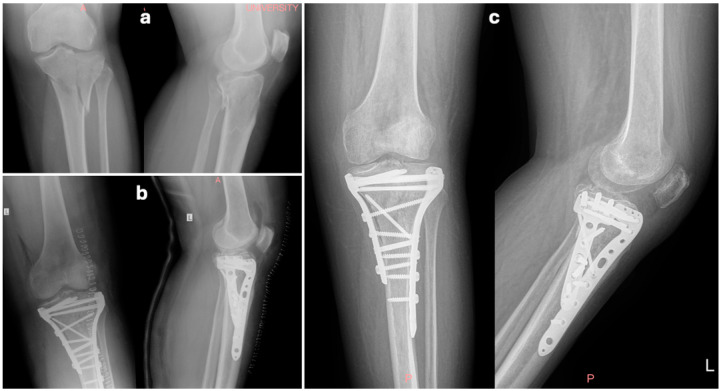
Preoperative X-rays of a 58-year-old female patient with a left Schatzker VI tibial plateau fracture (a). Post-operative X-rays show lateral and medial plating following reduction and grafting by an anterior midline approach (b). One-year follow-up X-rays (c) together with PROMs confirm a satisfying outcome.
